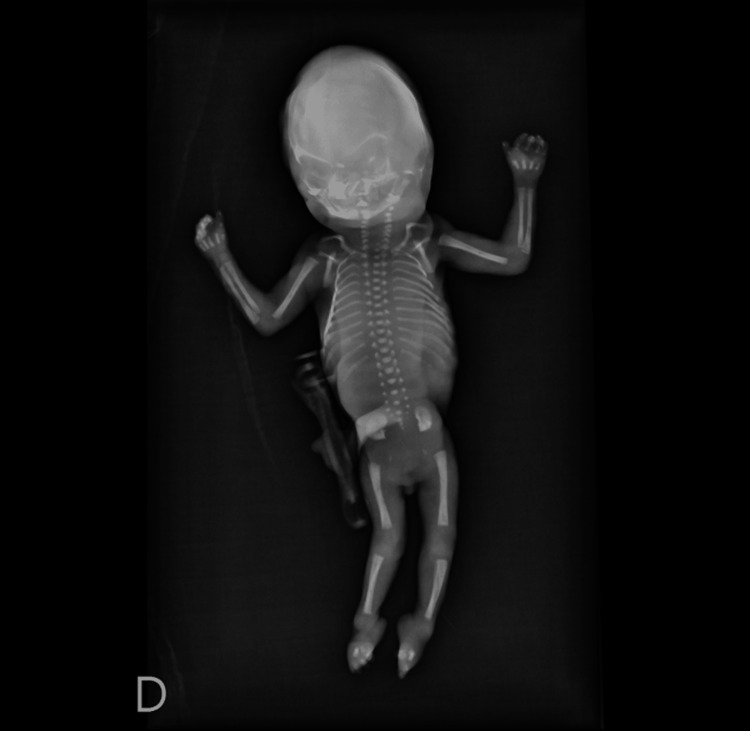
Skeletal radiographs No abnormalities, particularly no sacral defect, were present.
